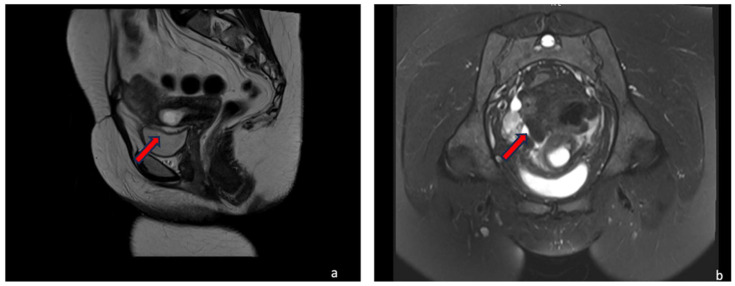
CSP Type I. Sagittal T2-weighted (a) and Axial (b) T2-weighted fat-saturation MRI sequences of the pelvis showed the gestational sac (red arrows) implanted in the lower anterior part of the uterine wall without any signs of interruption of serosa. A clear cleavage plane between the uterus and bladder is also shown.
